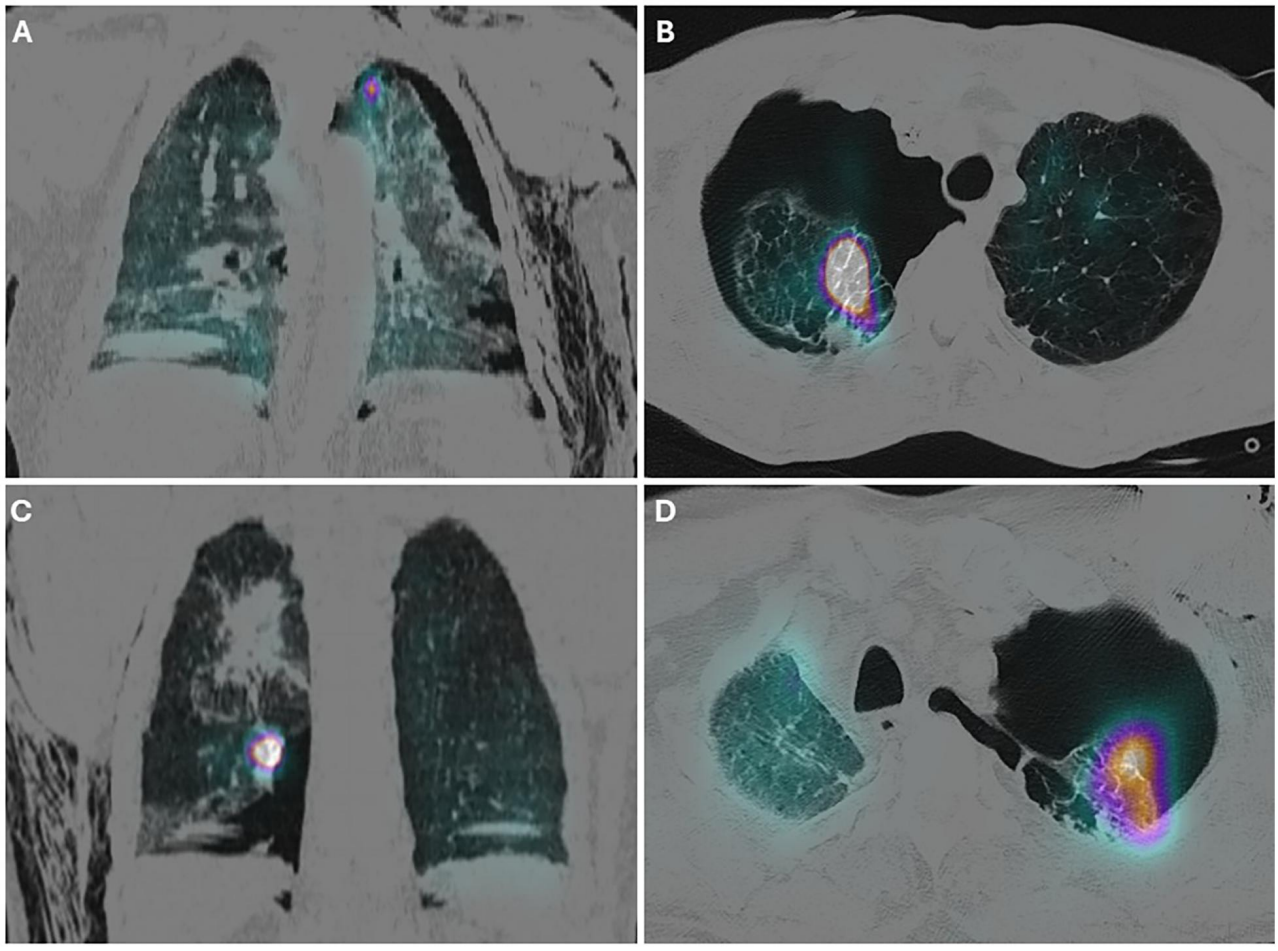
vSPECT/CT images showing coronal (A,C) and axial (B,D) slices from different patients, demonstrating leakage of 99mTc-technegas into the lung parenchyma.
